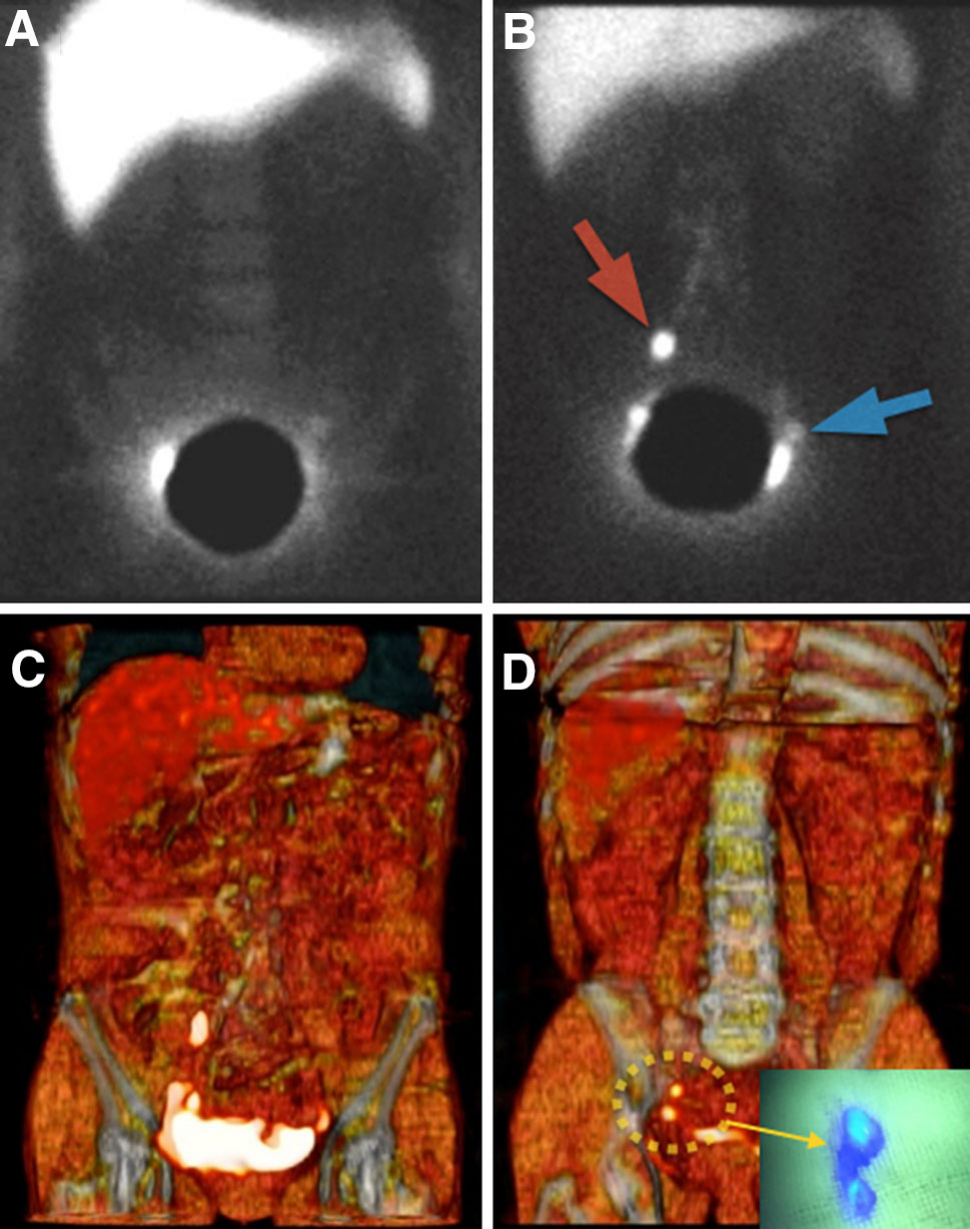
Endometrial cancer. Early planar image showed a very faint left node. A lied shield covered the injection area and high activity on the right side was supposed to be a partial zone of the injection area (a). Delayed planar image displays a right sentinel node (*red arrow*) and the previously observed left sentinel node (*blue arrow*). 3D volume-rendering image shows the same node distribution like b (c). A more detailed analysis of SPECT/CT data and 3D reconstructed images showed two posterior and caudal nodes (*dotted circle*) previous to the marked as sentinel node in b corresponding to external iliac nodes during surgery (*arrow*)
